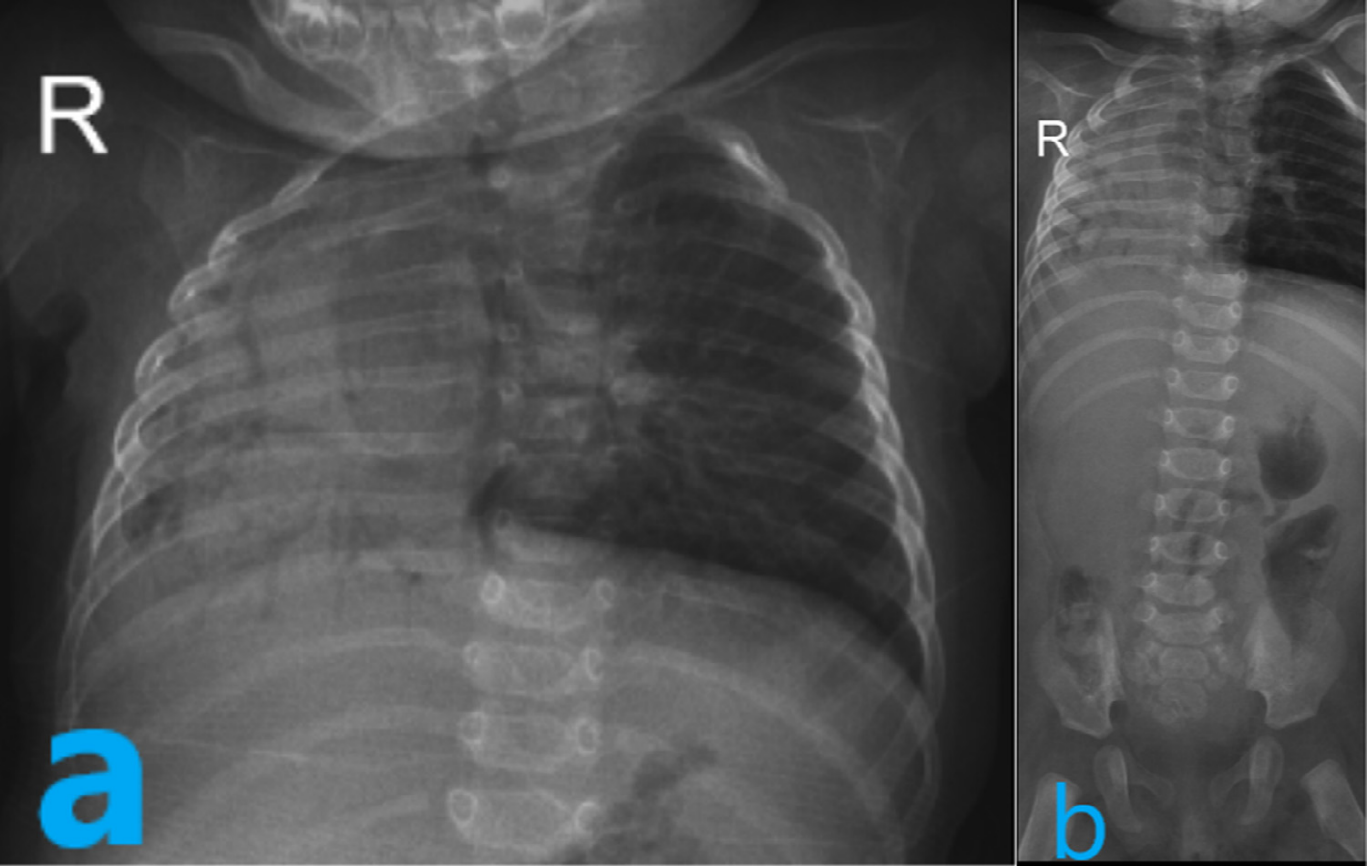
Chest X-ray (a) shows the atelectatic right lung contained air bronchograms with the abnormal position of the right main bronchus approximately at the base of the thorax. Evidence of hypoplasia of the sacrum was also observed in the thoracoabdominal X-ray (b).
